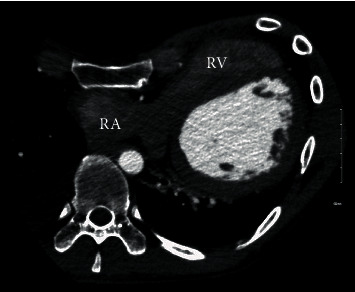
Computed tomogram showing pectus excavatum with severe compression and elongation of right atrium (RA) and right ventricle (RV).
